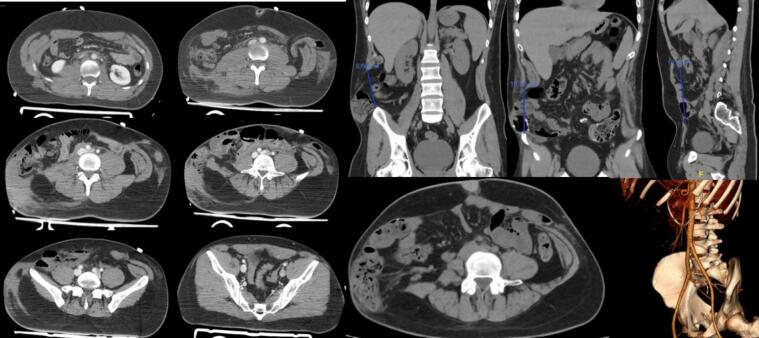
Emergency abdominal CT scan performed before urgent surgery. Vascular reconstruction.
